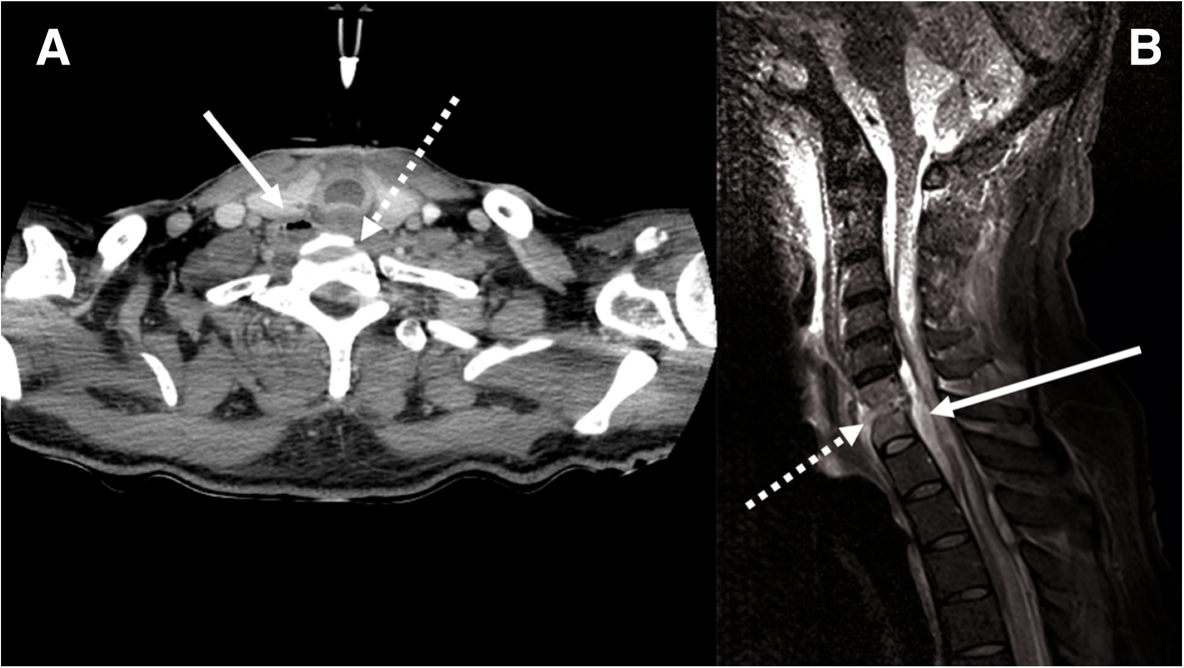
a Cervical computed tomography scanner: erosion of C6 vertebral body endplate (white dotted arrow) associated with epidural and right paravertebral hydro-aeric collections (white arrow). b Cervical and medullar magnetic resonance imaging: *STIR* hypersignal of disc with irregularity and erosion of C6-C7 vertebral body endplates (white dotted arrow), and epidural collections (white arrow)
